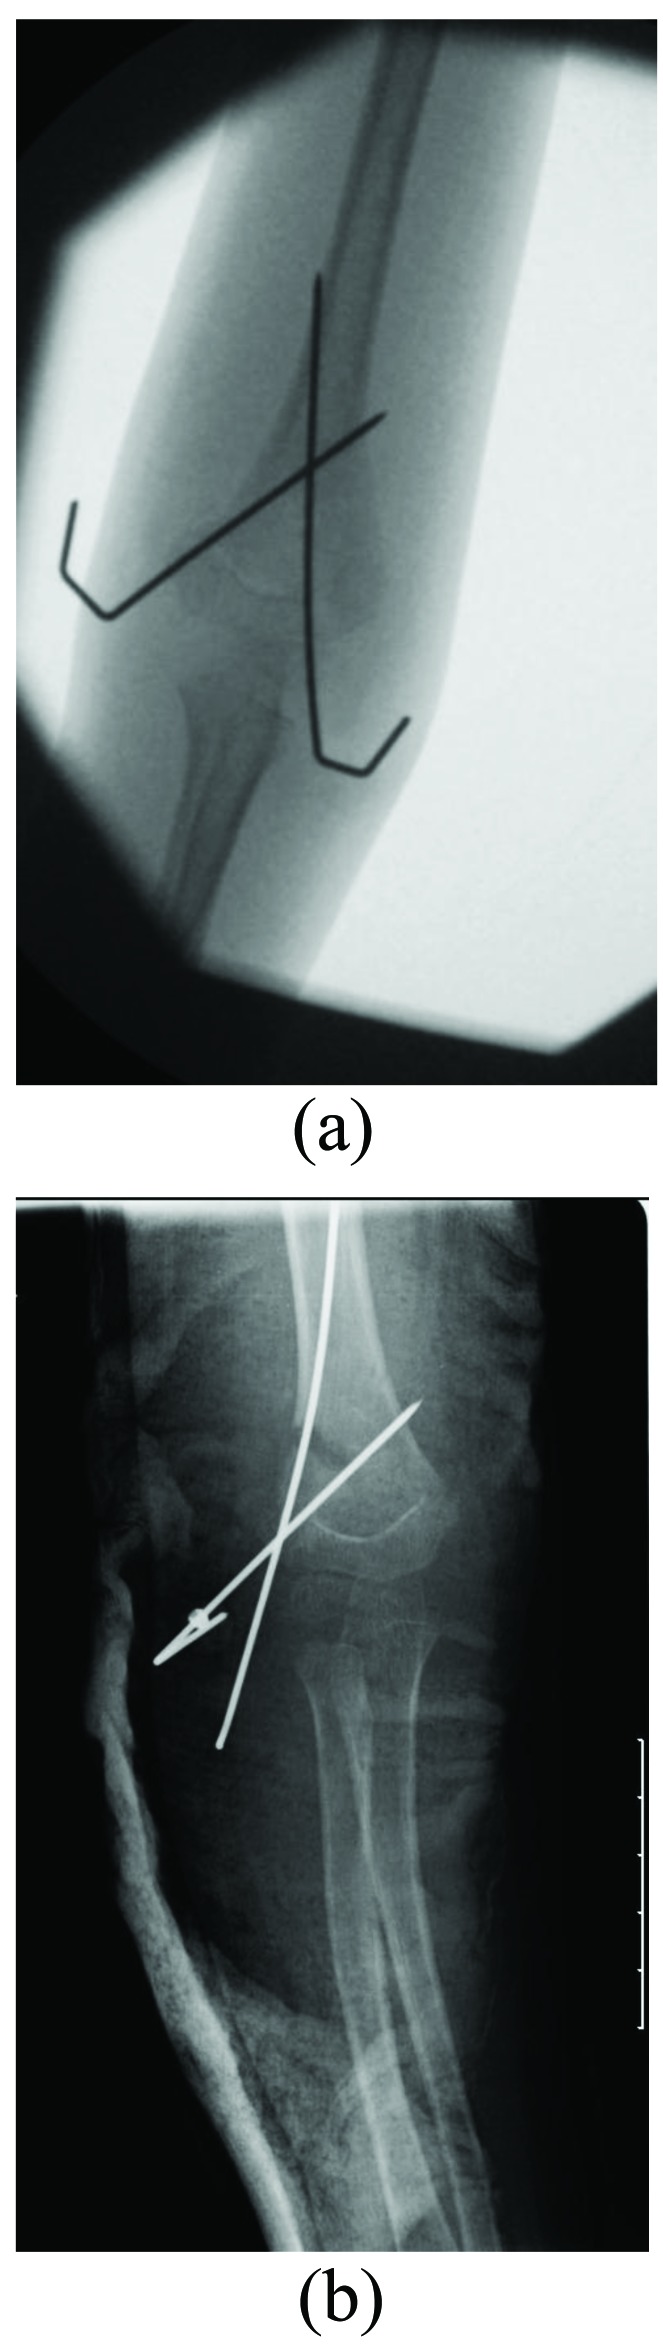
Radiographs showing the crossed (a) and lateral (b) K-wire configuration.
